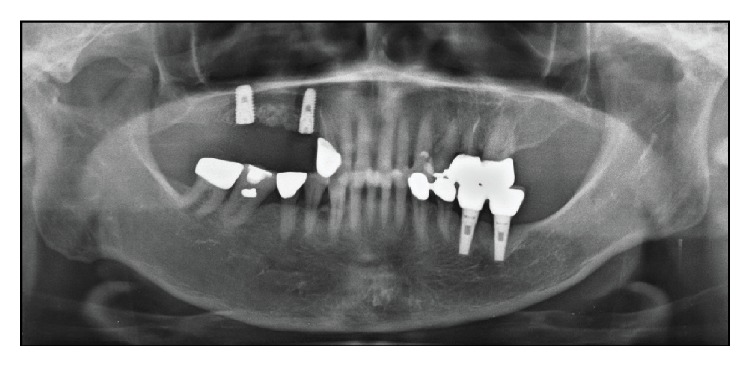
Postoperative radiographic view (panoramic X-ray image). Implant reinstallation on #14 and #16 regions four months after the implant removal.
